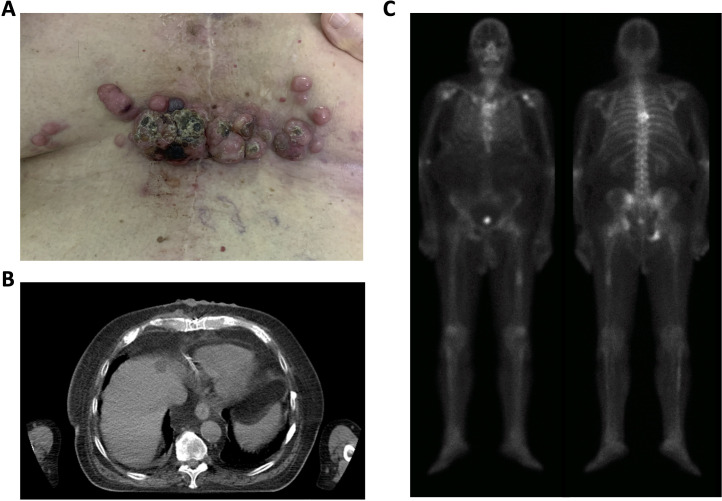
(A) Cutaneous lesions at diagnosis. (B) CT of skin nodules (C) Bone scan.
